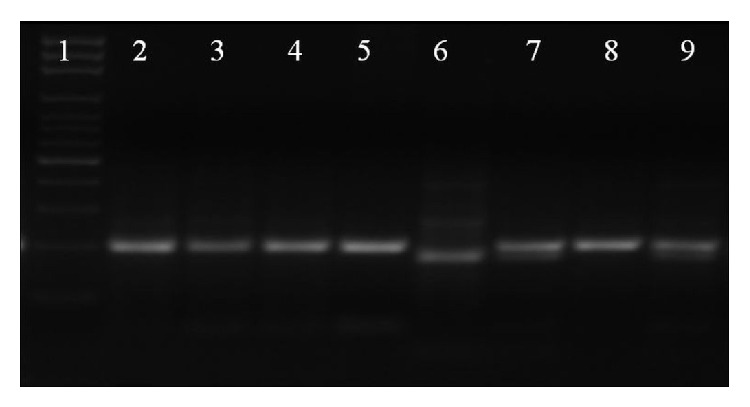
Image of agarose gel electrophoresis after* Hinf 1* restriction enzyme digestion. 1: 100 base pair DNA ladder. 2: PCR product, 3–5, 8: CC genotype, 6: TT genotype, 7, 9: CT genotype.
